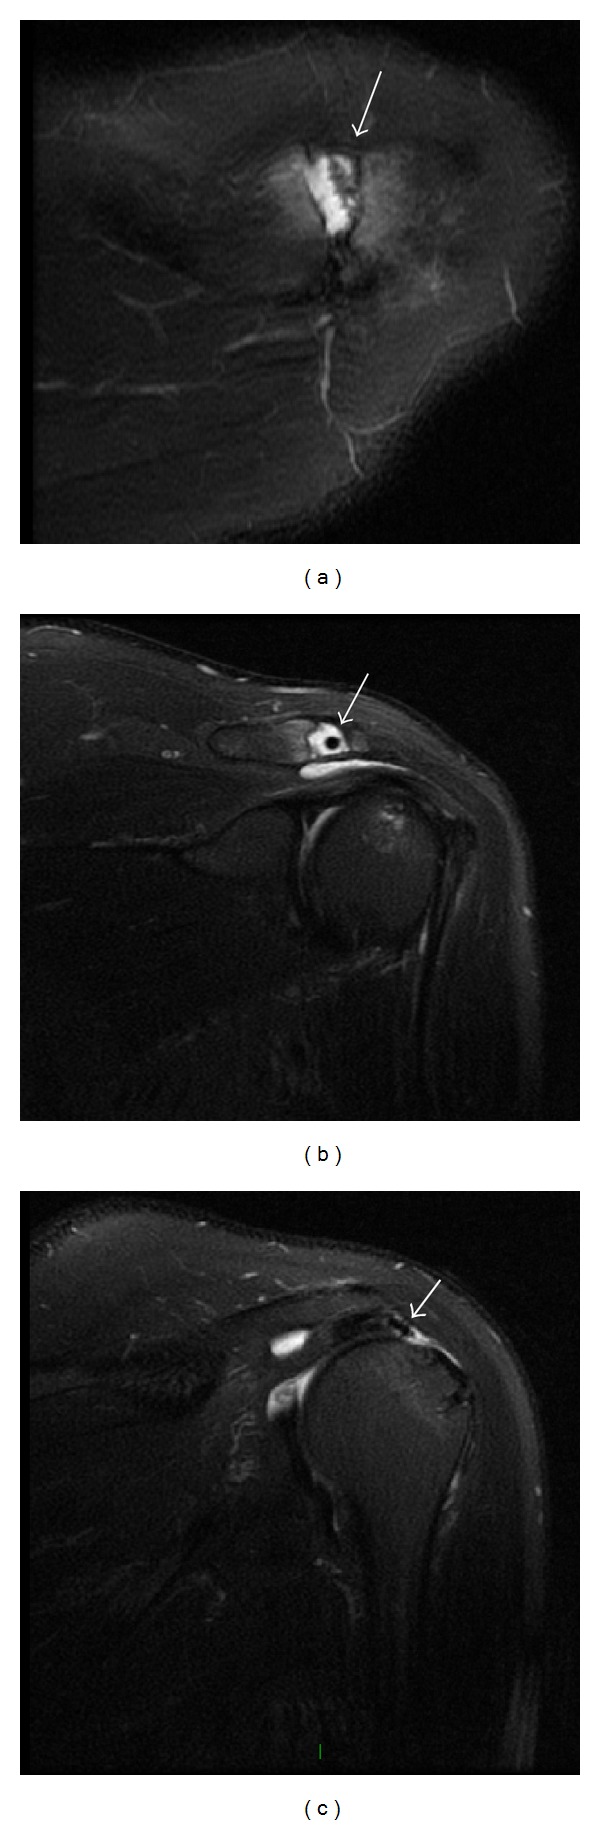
T2-weighted MRI of the left shoulder. (a) Axial view and (b) coronal view showing suture anchor located in the acromioclavicular joint (white arrow); (c) coronal view showing the suture anchor attached to the tendon stump of a retear of the rotator cuff (white arrow).
